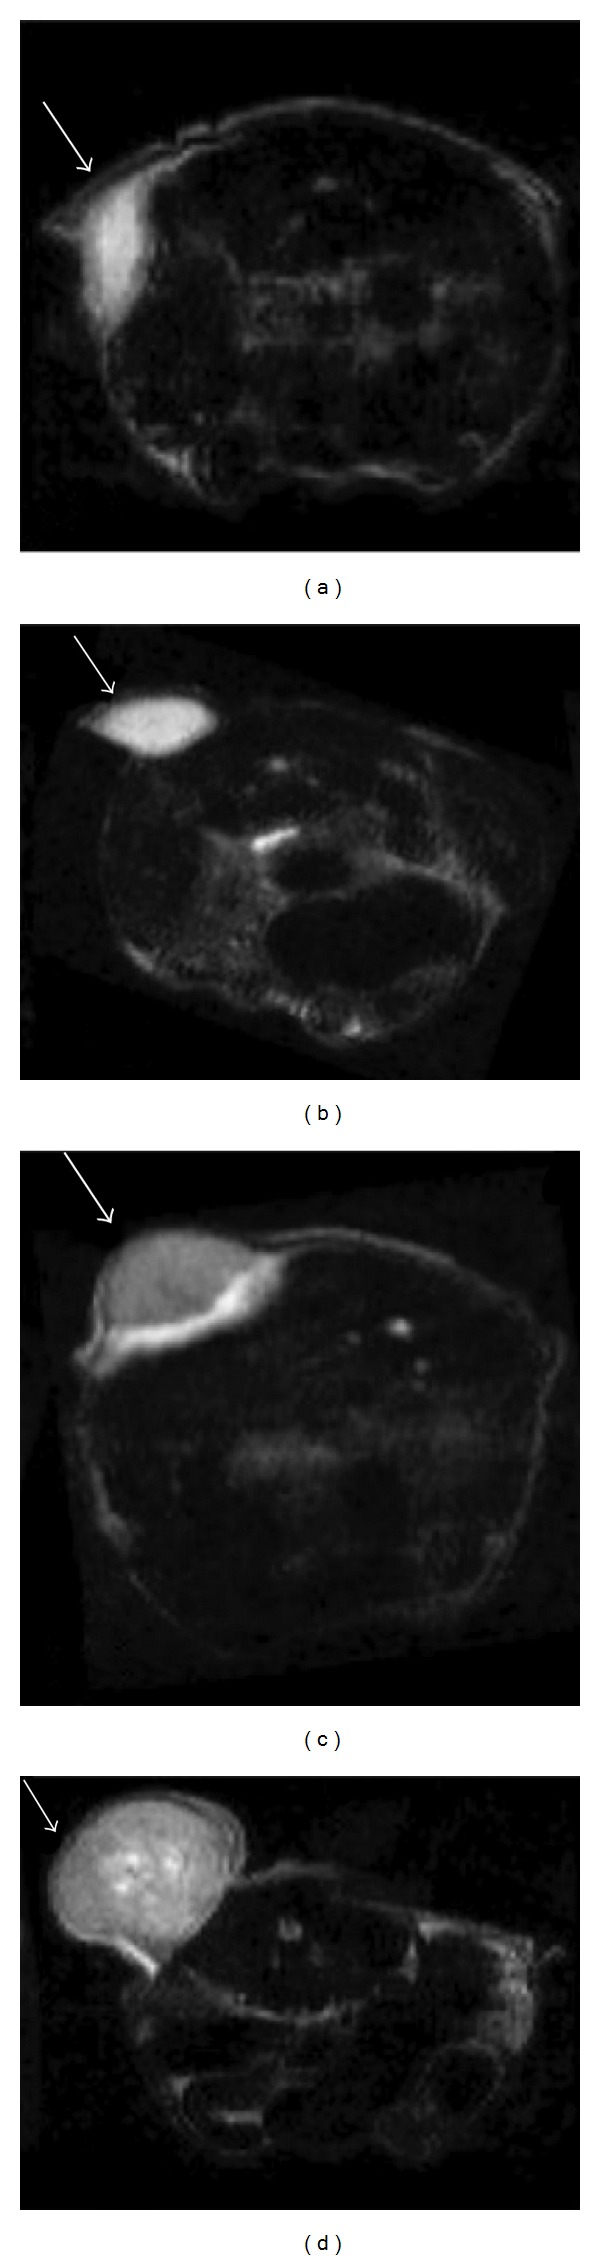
MRI imaging response to combination therapy in Ewing sarcoma. T2-weighted fast spin-echo images (TE/TR = 72/2400 ms) with a resolution of 312 mm demonstrating the tumor sizes in dasatinib (a), combination (b), triciribine (c), and untreated (d) at day 6. Representative datasets were chosen to reflect the overall trend of tumor size and growth. Lesions are indicated by arrows.
